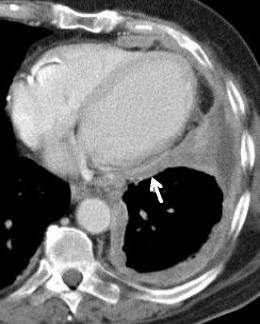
Stage III of MM; contrast-enhanced CT scan: axial view showing a circumferential pleural thickening, involving the mediastinal pleura and the pericardium (*white arrow*), classified as T3, N0, M0
